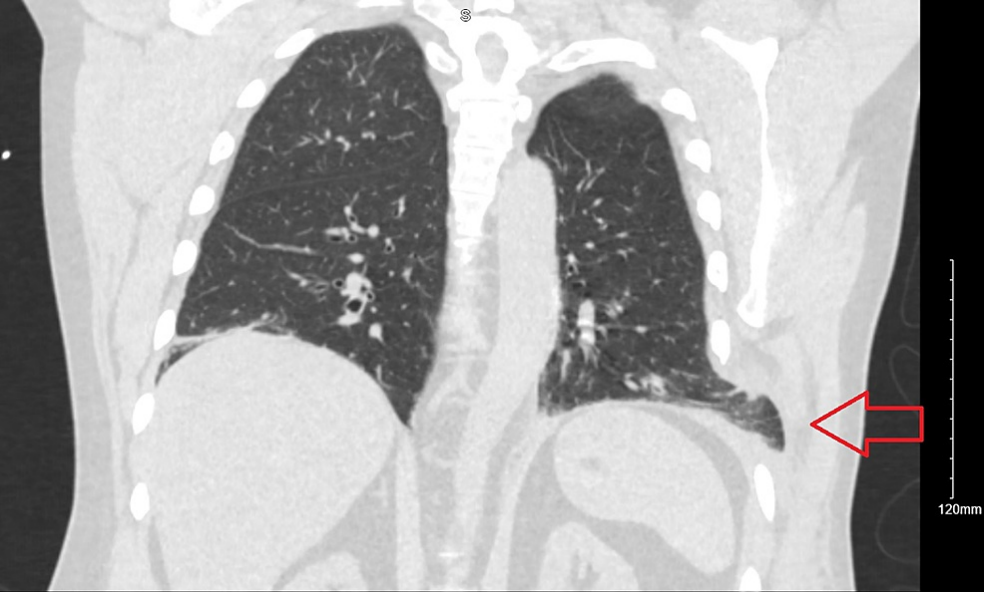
Coronal view of chest CT imaging highlighting area of lung herniation (red arrow) in left lower thoracic wall.
